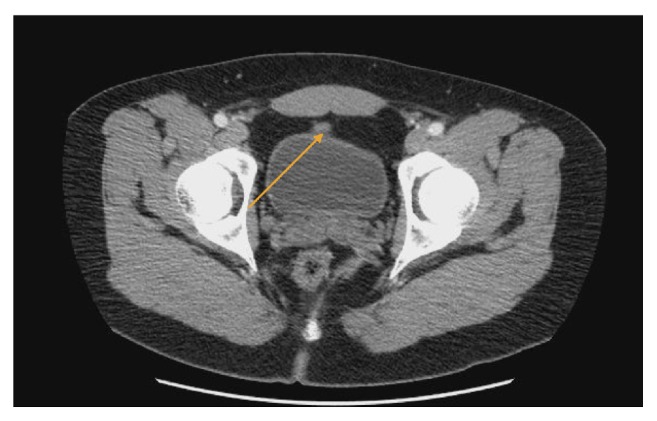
Computed tomography (CT) scan of the abdomen and pelvis axial section showing urachal remnant anterosuperior midline of the bladder (orange arrow).
